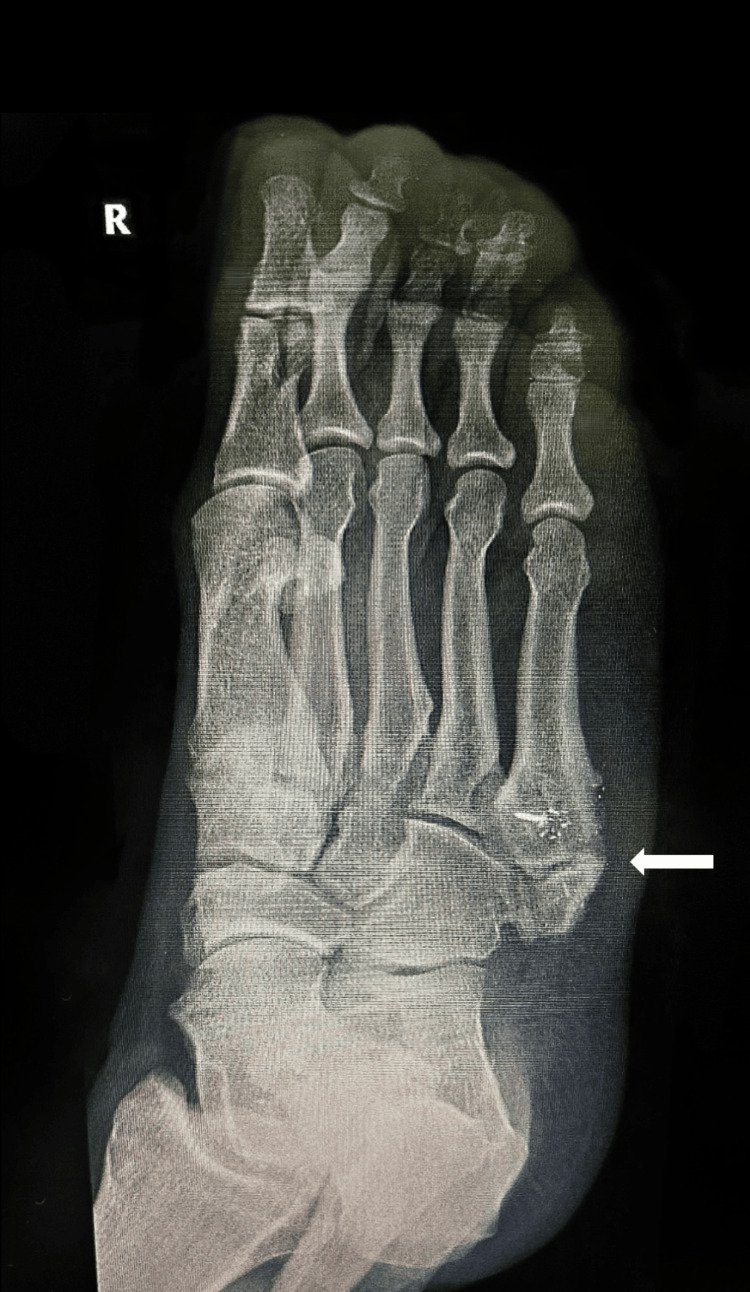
Ankle X-ray revealing the presence of an OVP bilaterally (right) OVP, os vesalianum pedis
